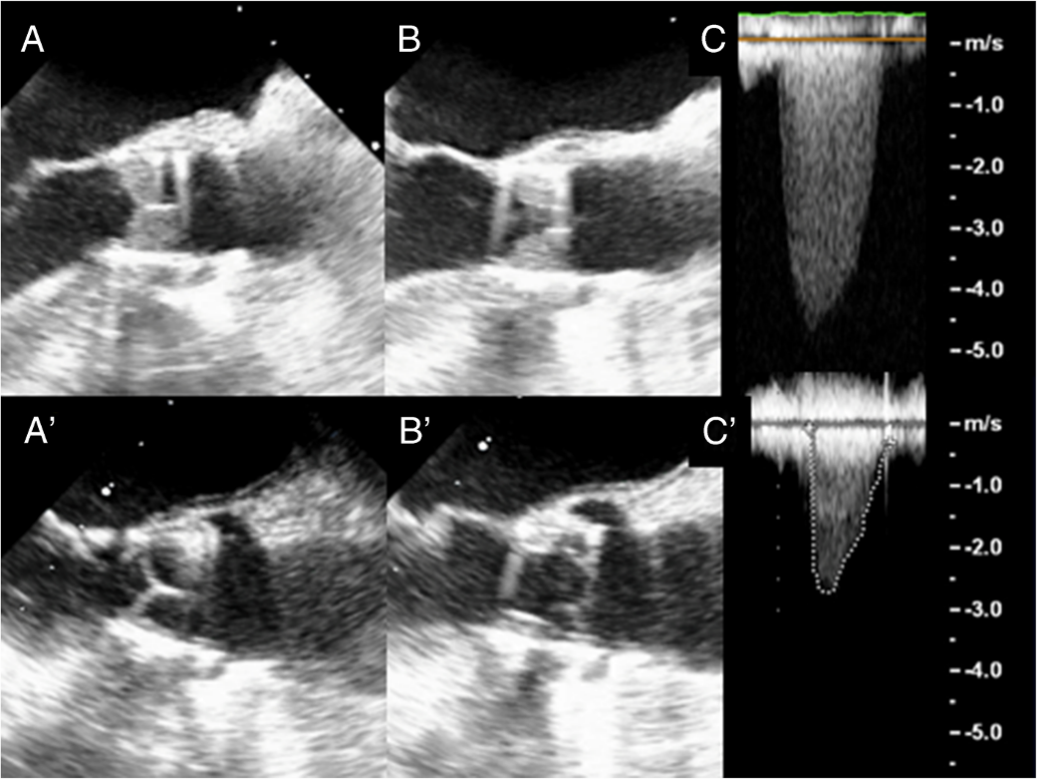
Transesophageal echocardiogram (TEE) of the aortic bioprosthesis in diastole (A) and in systole (B), with transvalvular continuous-wave Doppler (C) revealing a mean gradient (MG) of 64 mmHg and a Vmax of 5 m/s. Same TEE sections and continuous-wave Doppler (A’, B’ and C’) after 1 month of anticoagulant therapy: MG = 15 mmHg and Vmax = 2.7 m/s with unchanged heart rate and ejection fraction.
